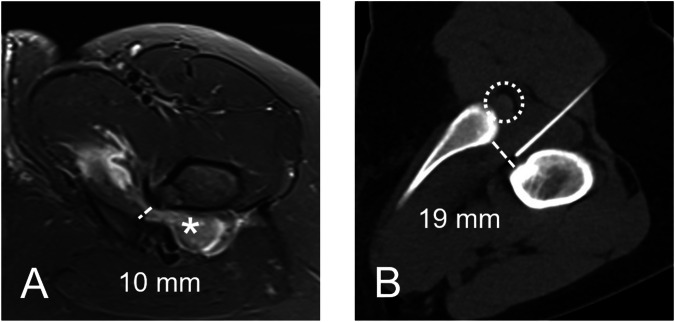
A Axial short-tau inversion recovery MR-image with a narrow IFS of 10 mm (dashed line) and concomitant QFM edema (asterisk) in the supine position and neutral leg rotation. B Axial CT-image during diagnostic IFS infiltration in the prone position and maximum internal rotation, allowing enlargement of the IFS to 19 mm (dashed line) and sufficient distance of the needle to the ischial nerve (dotted circle) during injection of the local anesthetic
